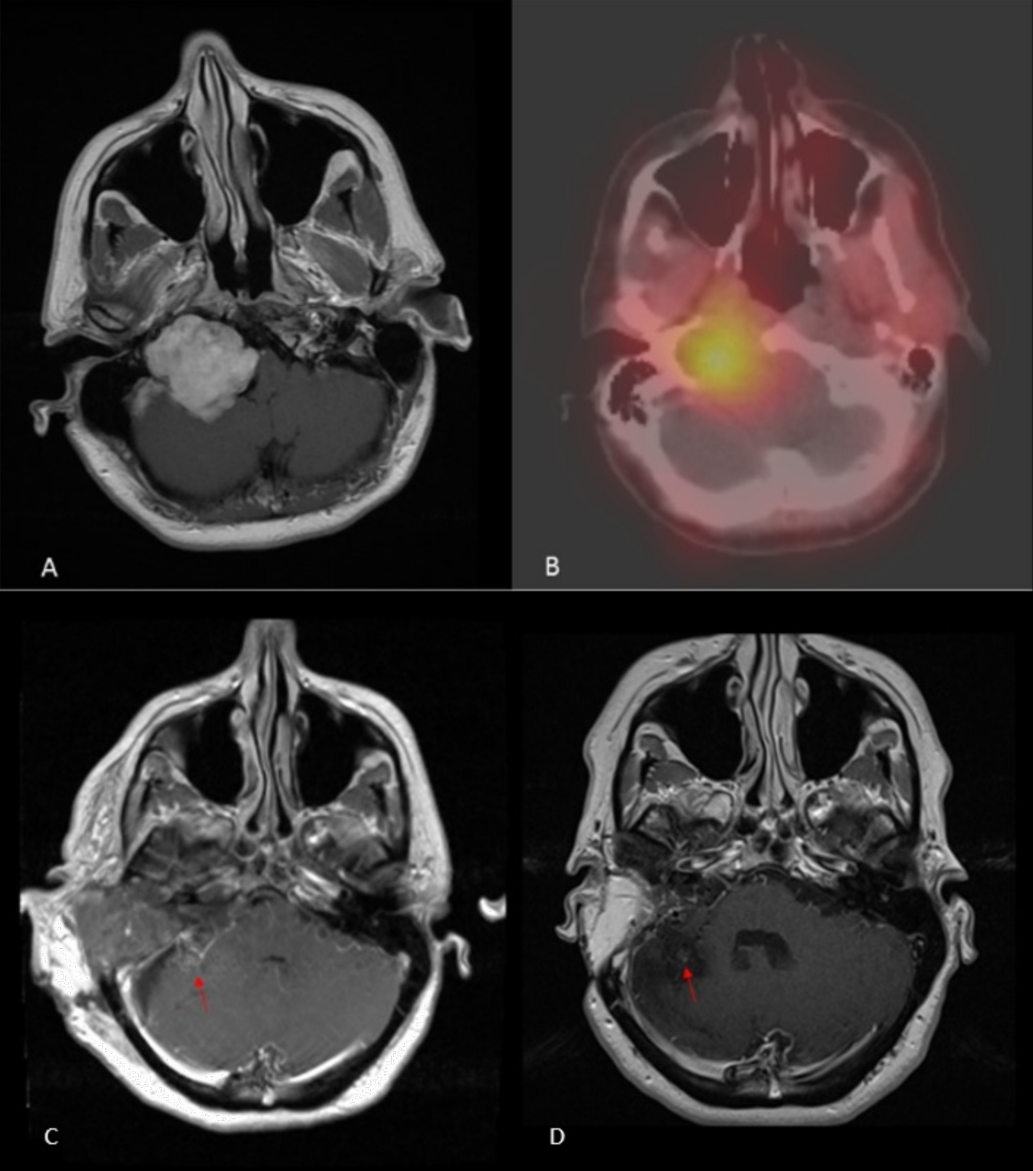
Magnetic resonance imaging and Octreoscan T1-weighted post-contrast MRI (magnetic resonance imaging) (A) demonstrates the avidly enhancing large right skull base mass centered at the jugular foramen, involving the right carotid space inferiorly and the right hypoglossal canal. 24-hour In-111 Pentetreotide SPECT-CT (single-photon emission computed tomography) fused images (B) demonstrates intense uptake in the mass. Initial post-operative T1-weighted post-contrast MRI (C) demonstrates expected post-surgical changes related to the skull base mass resection with no residual enhancing tumor. Follow-up MRI (D) four years later demonstrates stable post-operative changes with no residual mass. Tiny focus of enhancement beneath the tentorium likely reflected residual DAVF (dural arteriovenous fistula) (red arrow).
